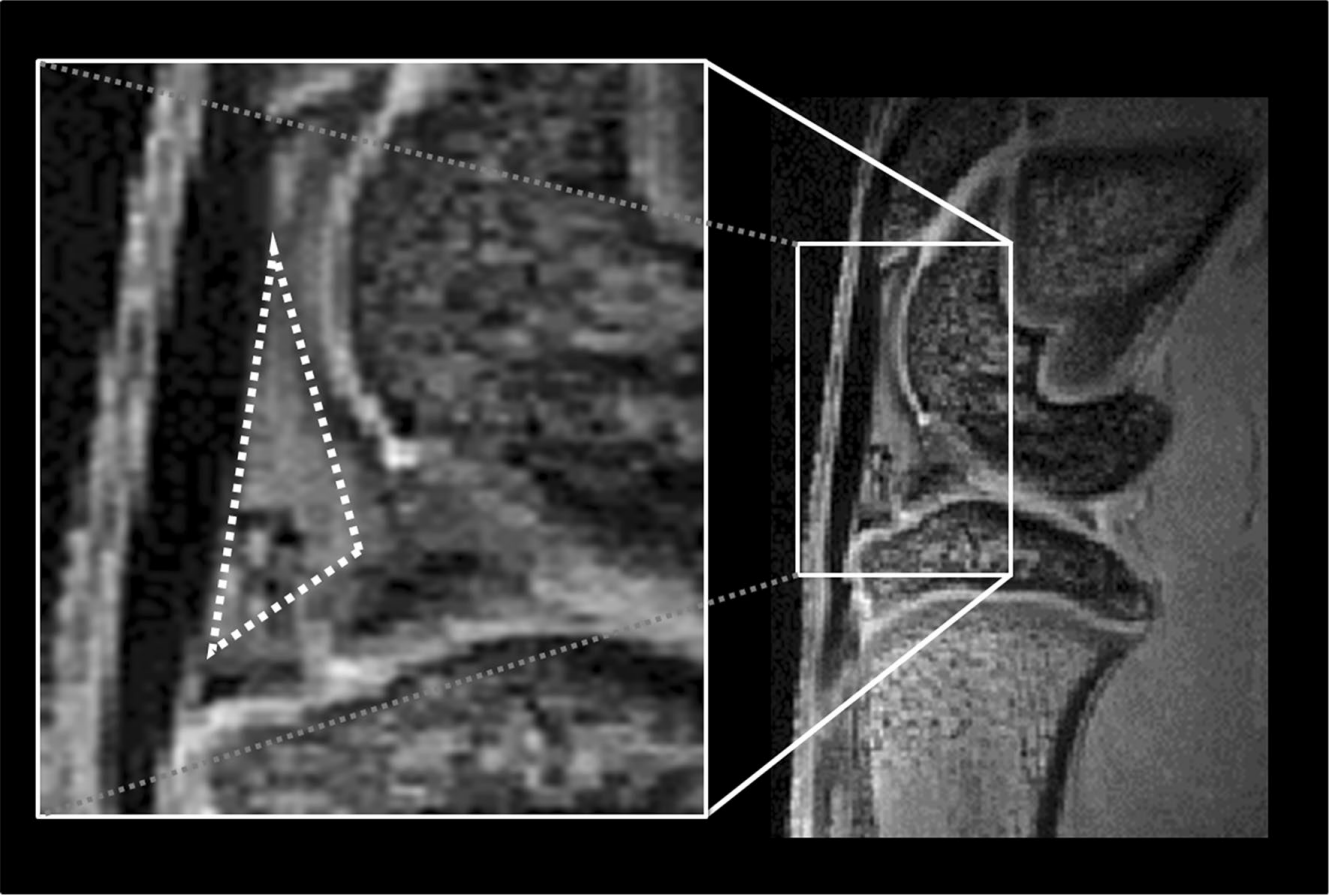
The selection of a ROI in the infrapatellar fat pad. The infrapatellar fat pad ROIs in DCE-MRI and MRI T2* were positioned using the sagittal image with the largest fat pad available. A triangular ROI under the infrapatellar tendon and near the anterior femur and tibial plateau was drawn manually without including the anterior horn of the meniscus of the knee joint.
